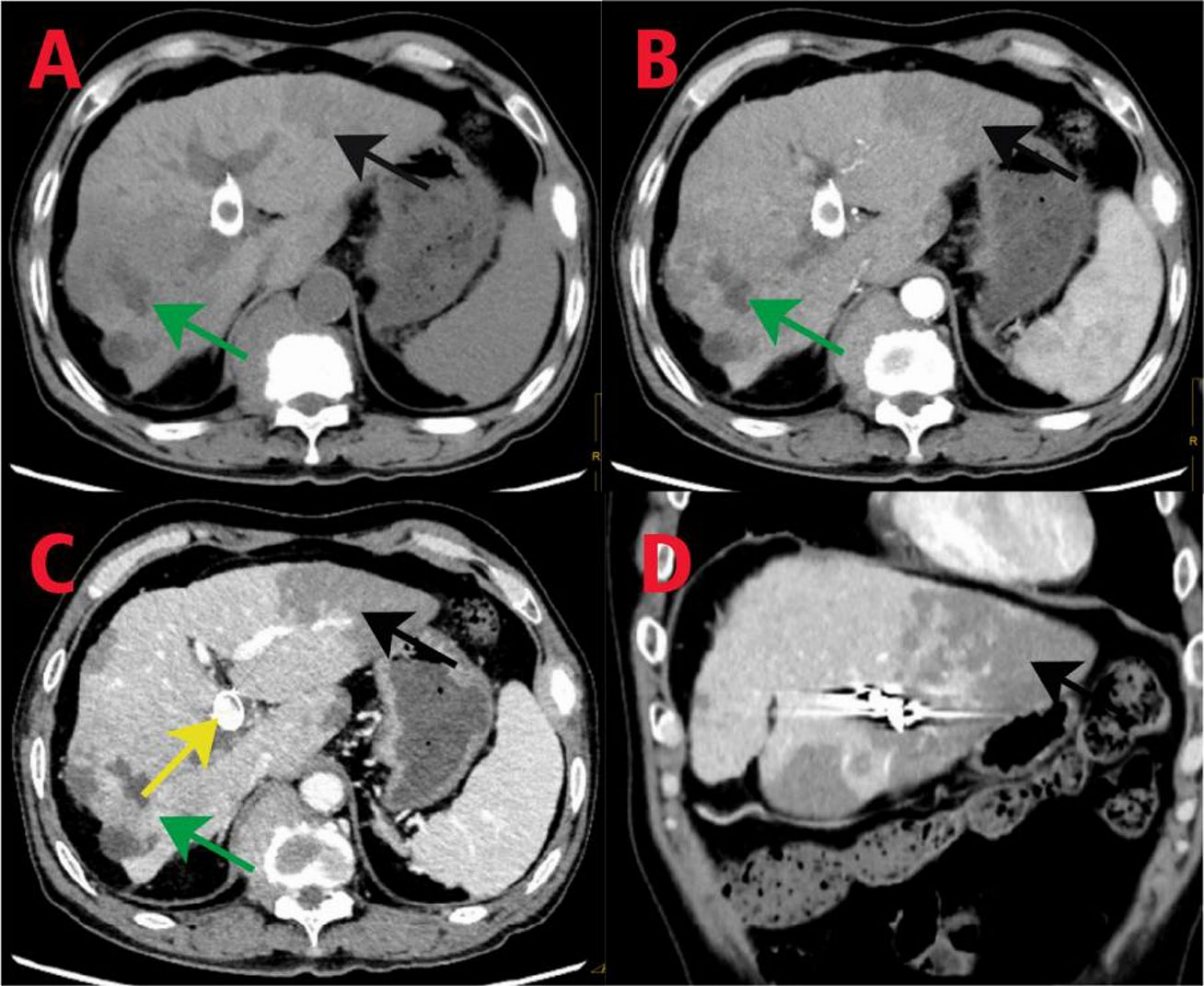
CT imaging findings: In the plain scan, a nodular low-density lesion with indistinct borders is observed in the right lobe of the liver (A, green arrow). Contrast-enhanced scan shows no significant enhancement in the arterial phase (B, green arrow), and reduced enhancement in the venous phase, with lower enhancement degree compared to the surrounding liver parenchyma. Patchy low-density lesions are visible within the lesion (C, green arrow). Multiple patchy and slightly low-density lesions are observed within the liver parenchyma, with no significant enhancement on the contrast-enhanced scan. The previously enhanced lesion within the liver shows no obvious arterial blood supply. A patchy dense shadow is seen in the left lobe of the liver (A, B, C, D, black arrow). In the portal vein phase, a crescent-shaped filling defect is observed (reduced compared to previous scans) (C, yellow arrow)
